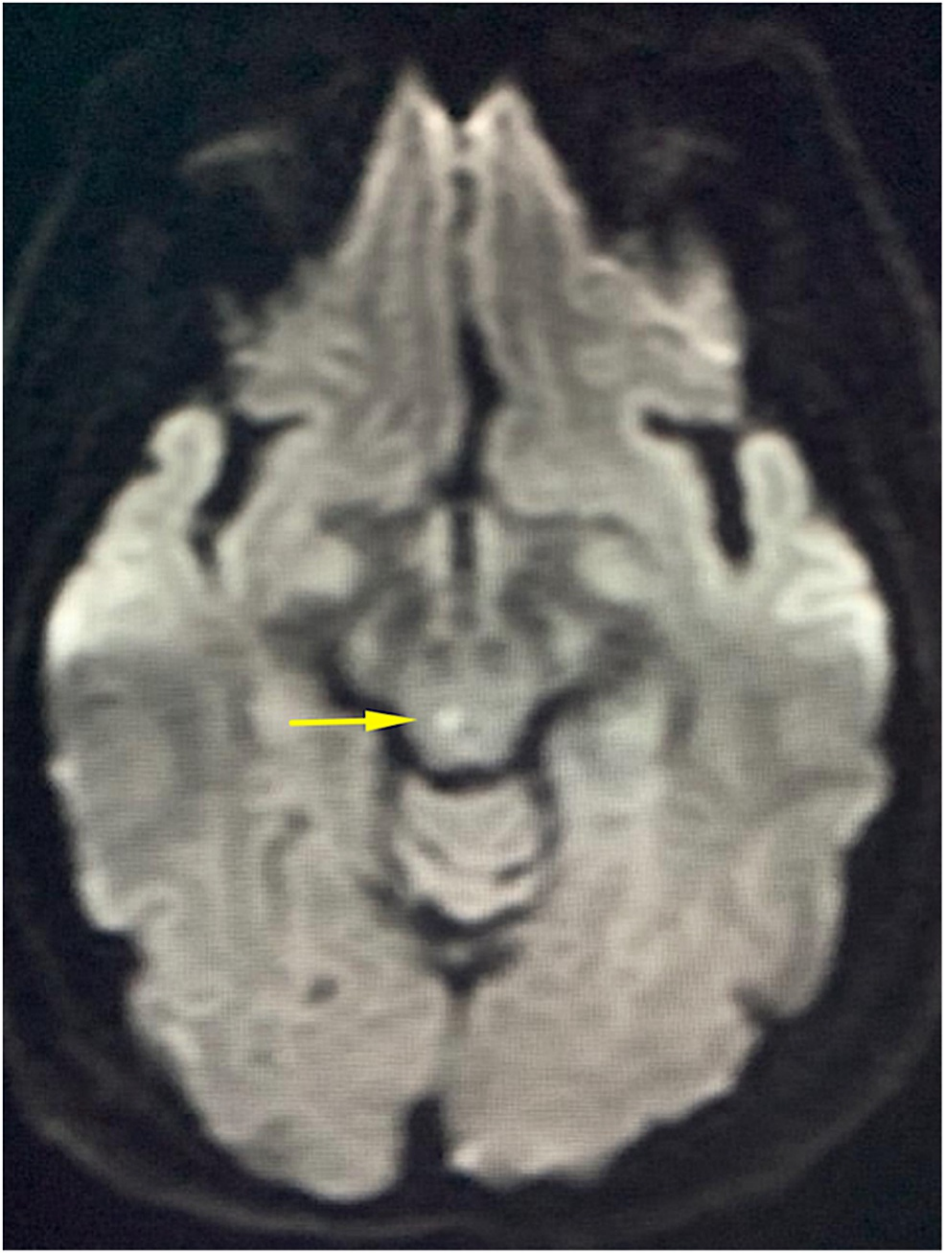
DWI axial view displaying a midbrain intensity DWI: diffusion-weighted imaging
